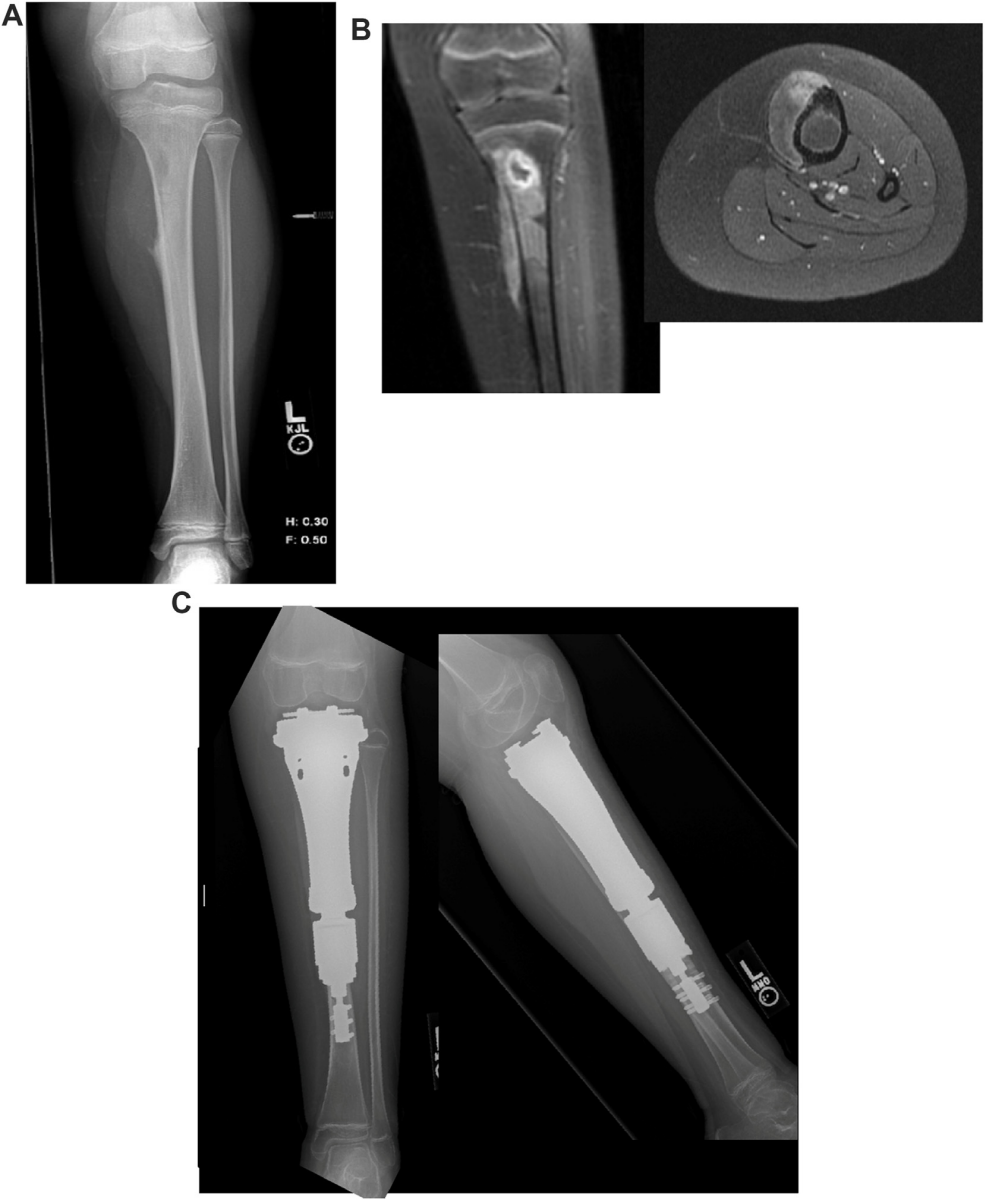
A. AP radiographs of an eight-year-old female with proximal tibia osteosarcoma. B. Post-gadolinium MR coronal and axial images obtained after administration of neoadjuvant chemotherapy showing residual disease. C. Postoperative AP and lateral radiographs following reconstruction with the custom proximal tibia hemiarthroplasty implant. AP, anteroposterior; MR, magnetic resonance.
